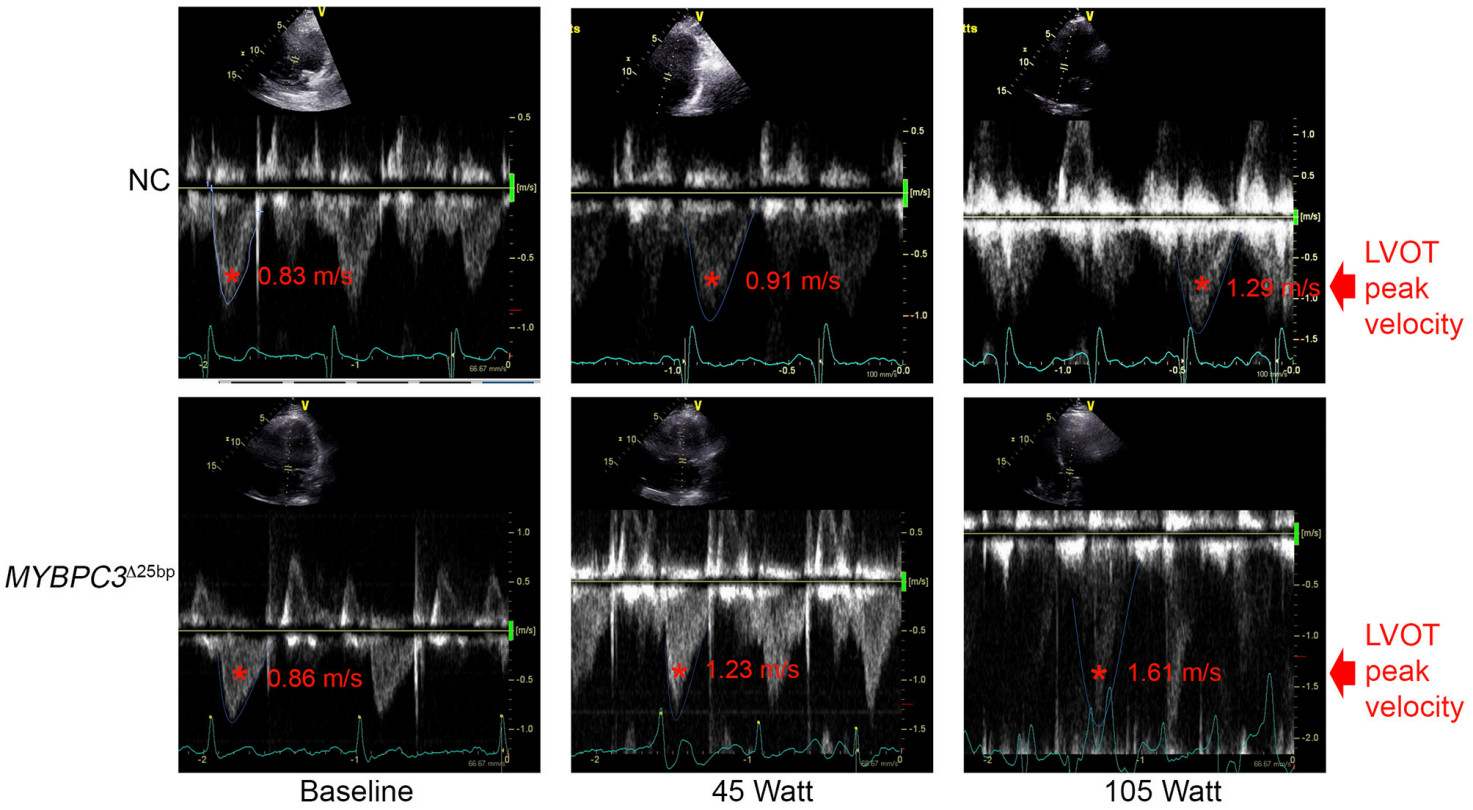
Spectral Doppler data depict LVOT peak velocity. Representative apical five-chamber view at baseline, 45 (W), and 105 (W) in a NC (upper panel) subject and a *MYBPC3*^Δ25bp^ variant carrier (lower panel). NC, non-carriers.
